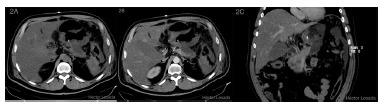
A) Axial unenhanced abdominal CT with no enhancement of body and tail of the pancreas; B) axial enhanced abdominal CT with no enhancement of body and tail of the pancreas; C) coronal enhanced abdominal CT with thickened unenhanced gastric wall and collection.
